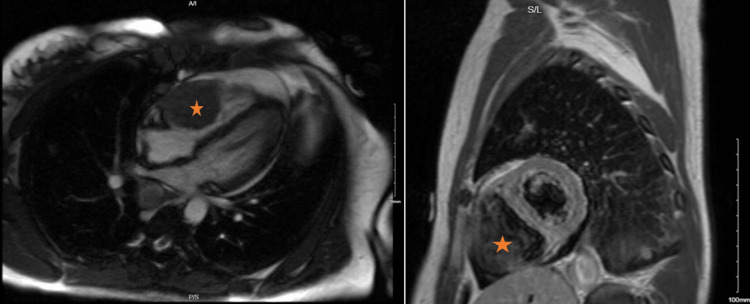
Cardiac magnetic resonance imaging. The image shows a large irregularly shaped mass measuring up to 4.0 × 3.3 cm and extending from the mid-RV free wall to the base of the RV and partially protruding into the RA during systole with imaging characteristics of a tumor (asterisk). RV: right ventricle; RA: right atrium
